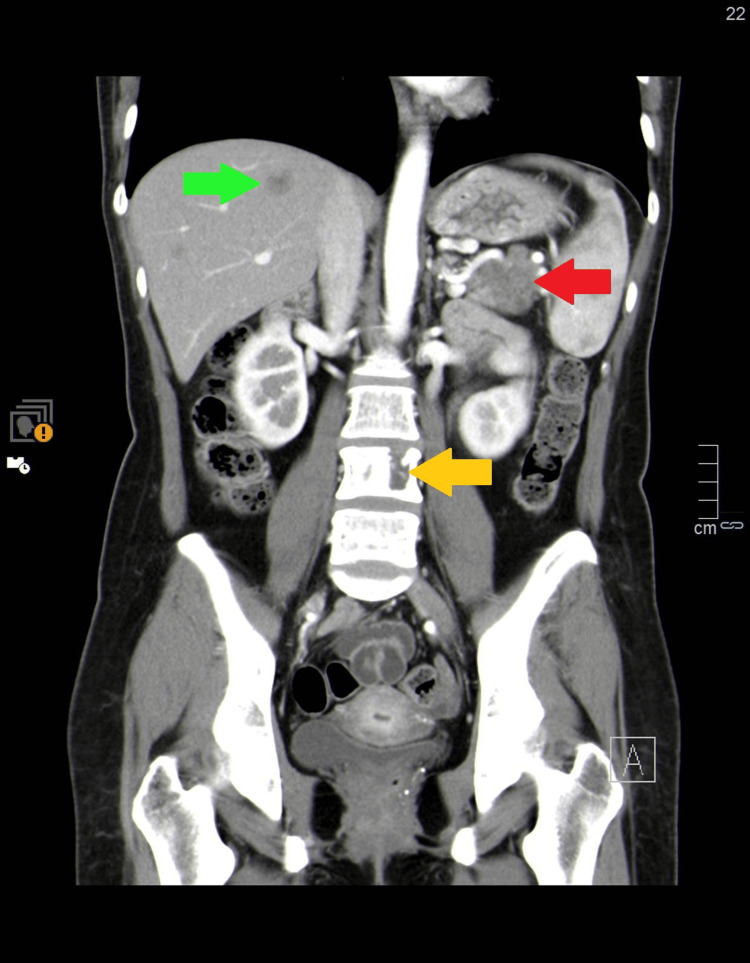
CT scan of the thorax, abdomen and pelvis showing one lesion in the distal pancreas (red arrow), one hepatic nodular lesion suggestive of metastasis (green arrow) and one osteolytic lesion in the L4 vertebral body (yellow arrow).
